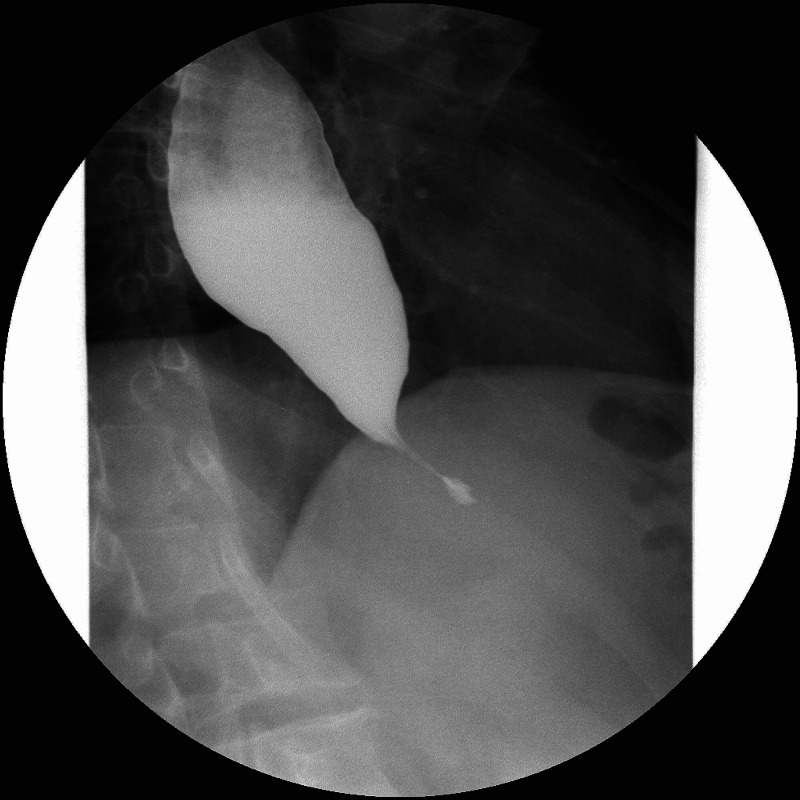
Contrast esophagram Upper gastrointestinal tract radiography with barium contrast showed a dilated esophagus with a tapered narrowing of the lower end – bird's beak appearance
